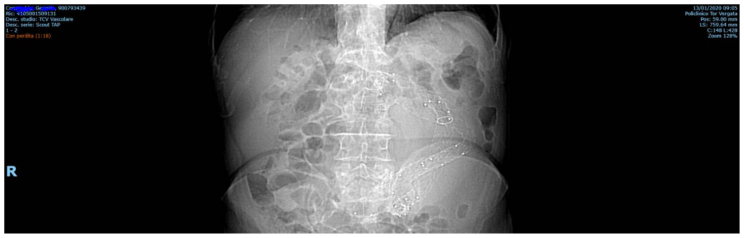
Direct X ray. Postoperative surveillance demonstrating the previous tortuosity. In the same period, computed tomography scan demonstrated no sign of endoleak.
